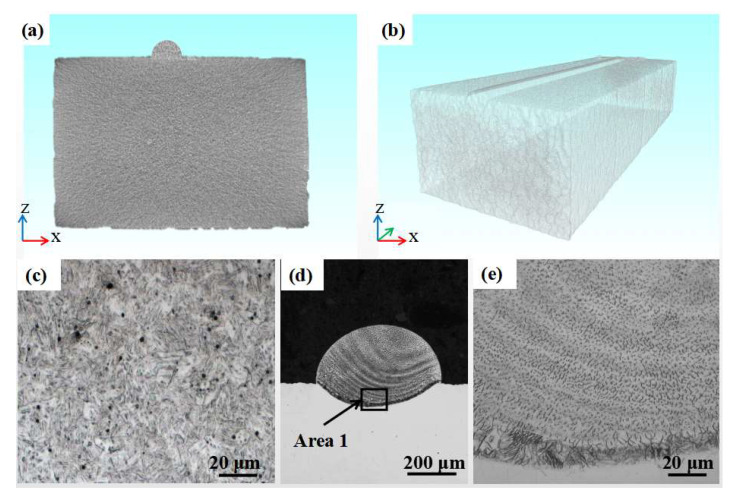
Internal quality: (a) CT scan image at depth of 12 mm, (b) transparent view obtained from CT scan analysis of printing layer, (c) micro-morphology of original wire, (d) micro-morphology of printing layer cross-section, and (e) enlarged view of Area 1.
